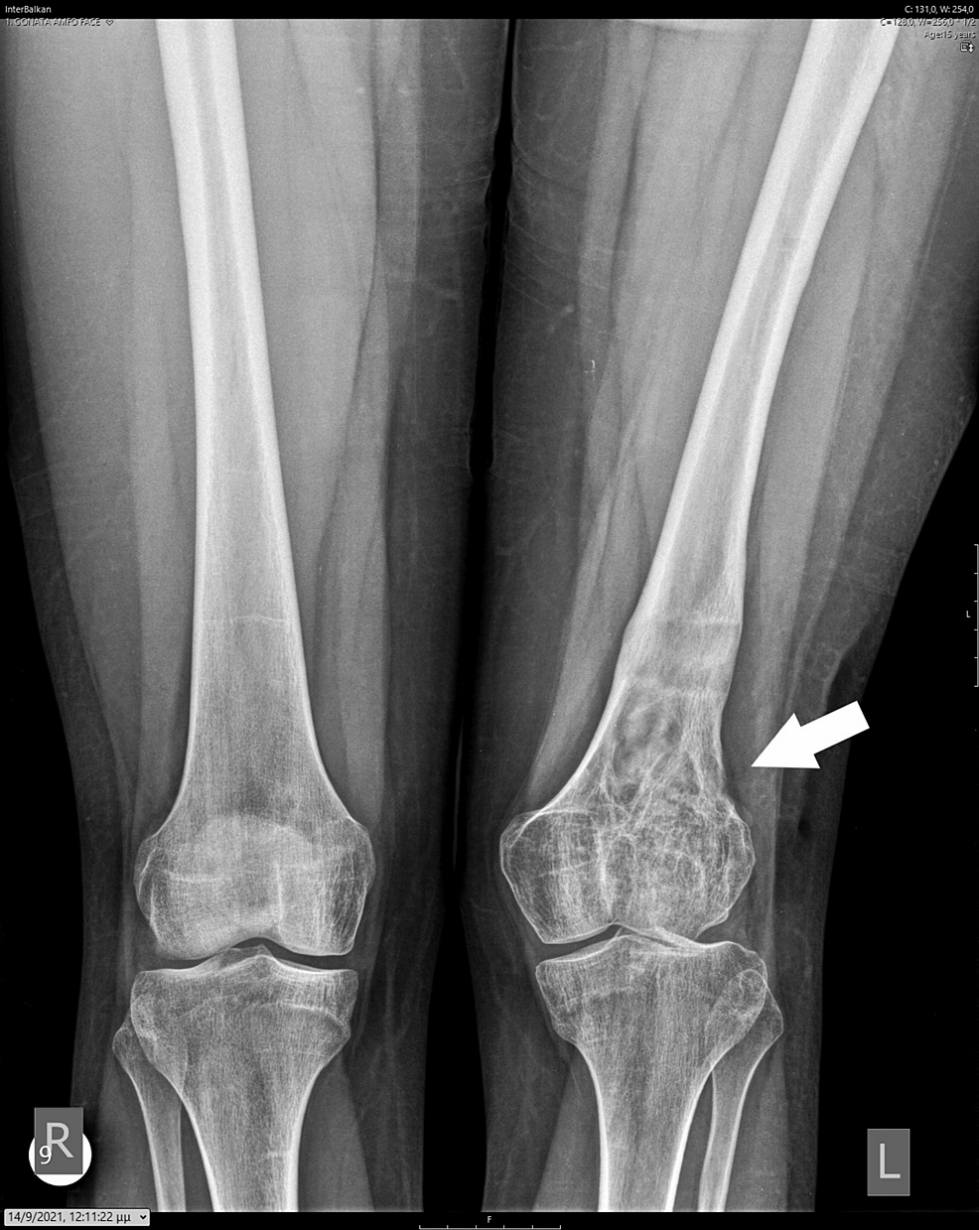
AP X-ray in the standing position, taken one year after the removal of the Ilizarov device, displays adequate bone formation with a residual LLD of 1 cm. AP: Antero-posterior; LLD: Leg length discrepancy.
